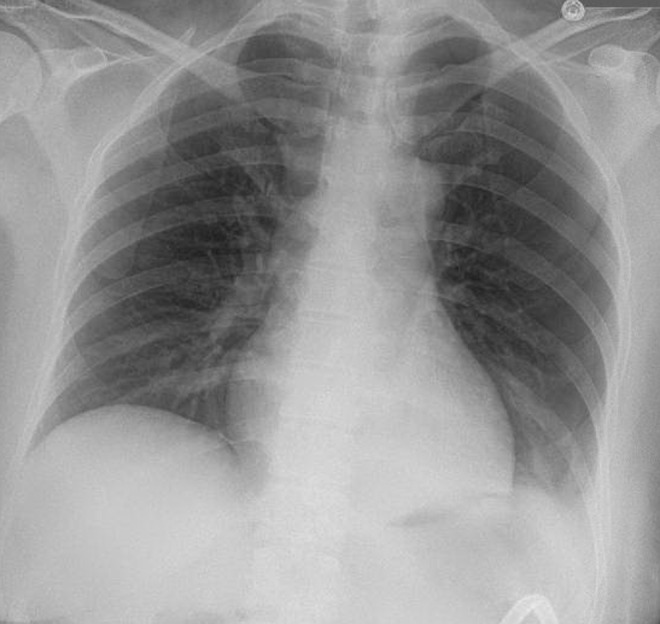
Postoperative day 1 chest radiograph reveals large left pneumothorax.
